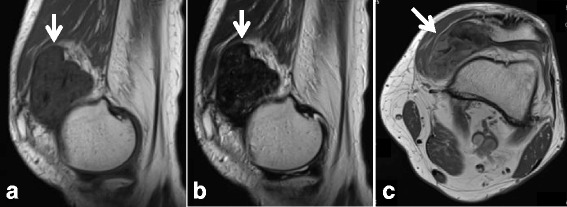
MR images of Case 3. a On T1-weighted images, the lesion (arrow) is heterogeneously isointense and hypointense compared to muscle. b On T2-weighted images, the lesion (*arrow*) is heterogeneously hypointense. c On contrast-enhanced T1-weighted images, the lesion (*arrow*) shows mild and patchy contrast enhancement
